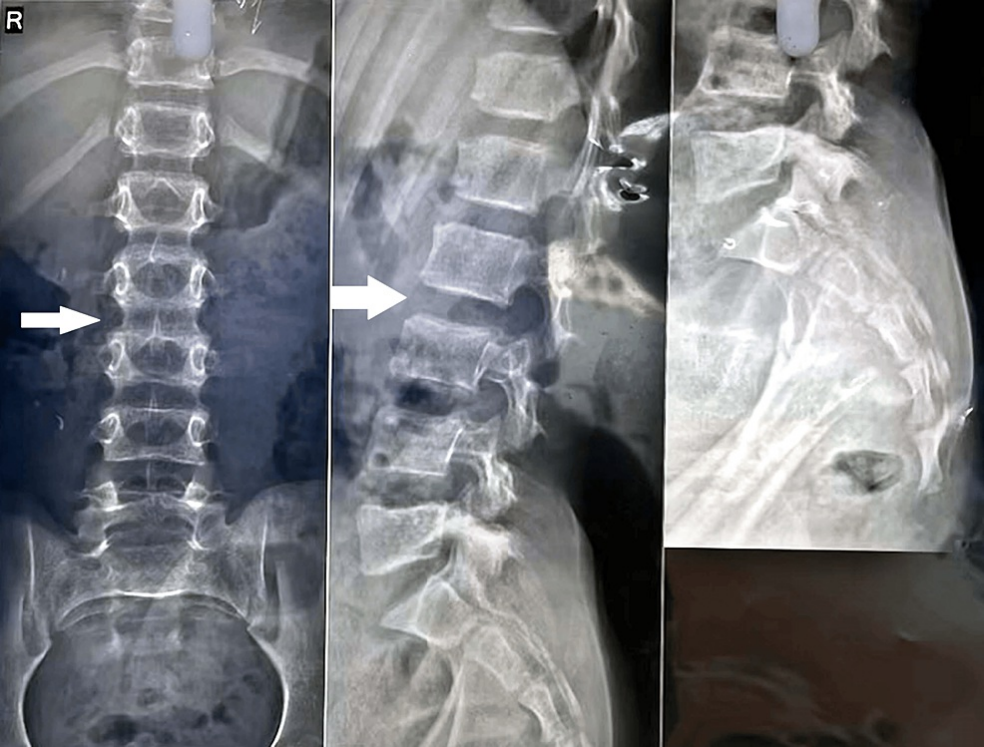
X-ray spine shows increased intervertebral disc spaces
